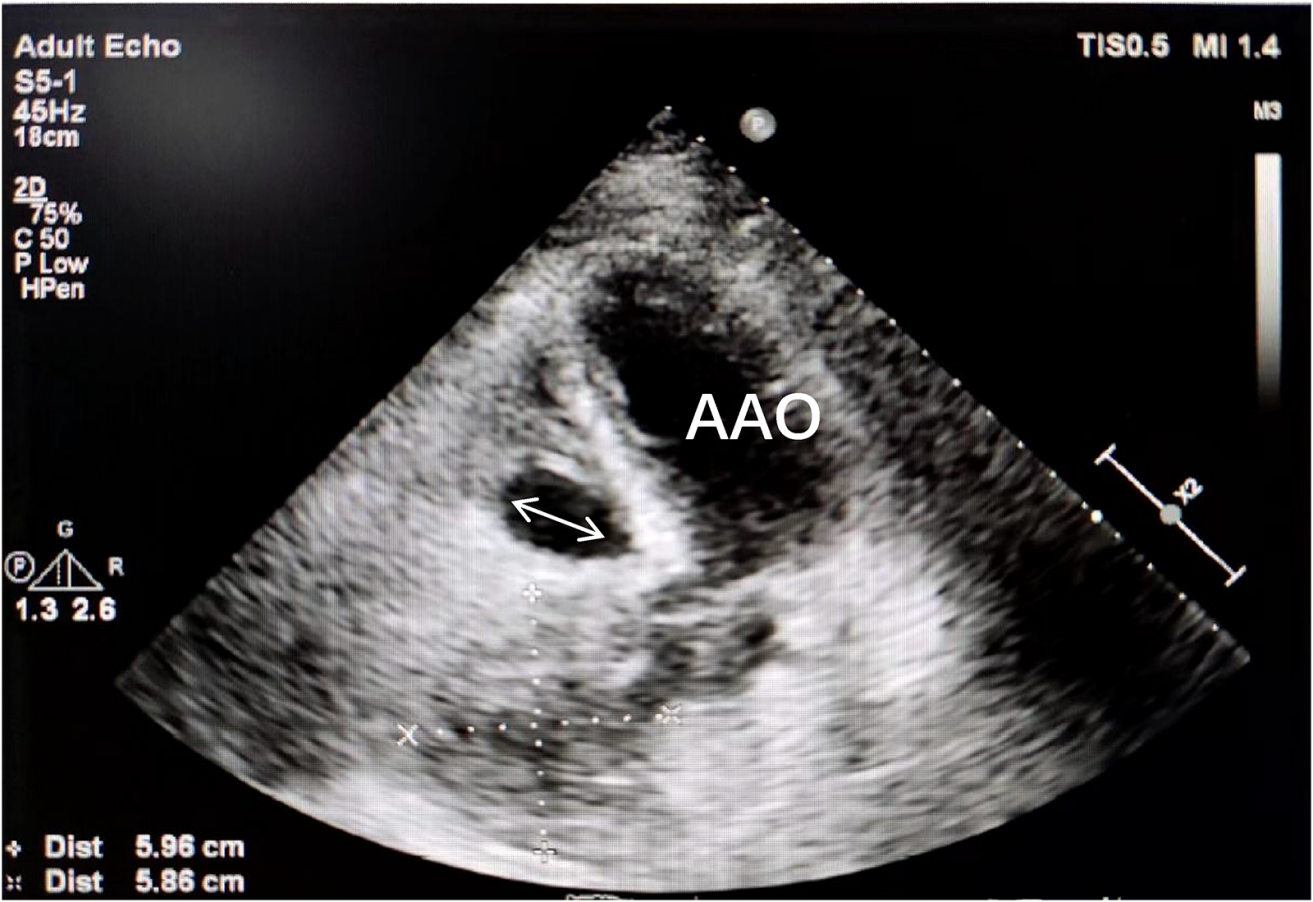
Transthoracic echocardiography (TTE) demonstrated continuous echogenicity along the wall of the aortic sinus to the right coronary artery, indicative of thrombosis within the residual lumen (white arrow) of the right coronary artery aneurysm.
